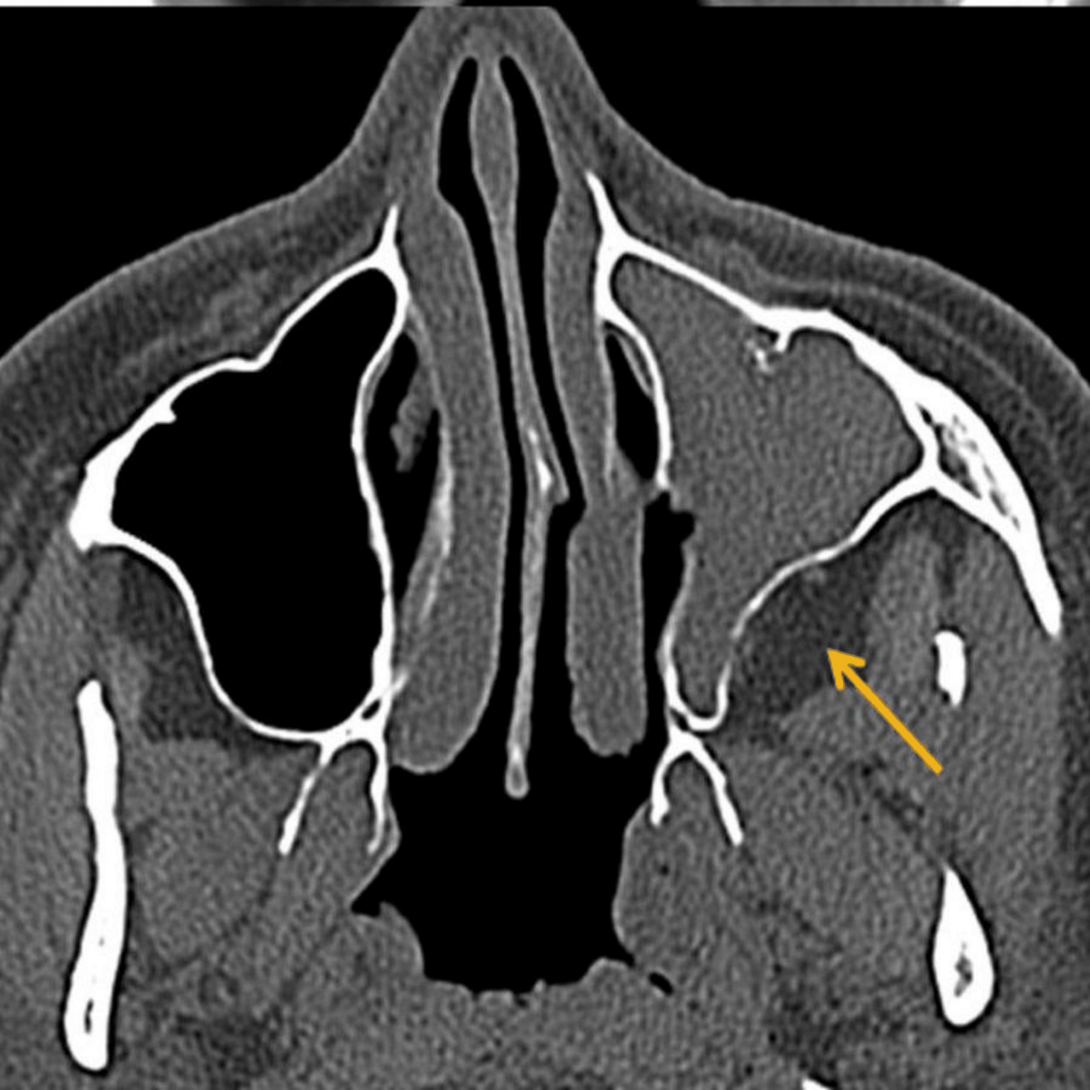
CT paranasal sinus of case 1. CT PNS (yellow arrow) showed isolated soft-tissue opacification of the right sphenoid sinus, without evidence of bony erosion, intracranial extension, or orbital invasion. PNS: paranasal sinuses.
